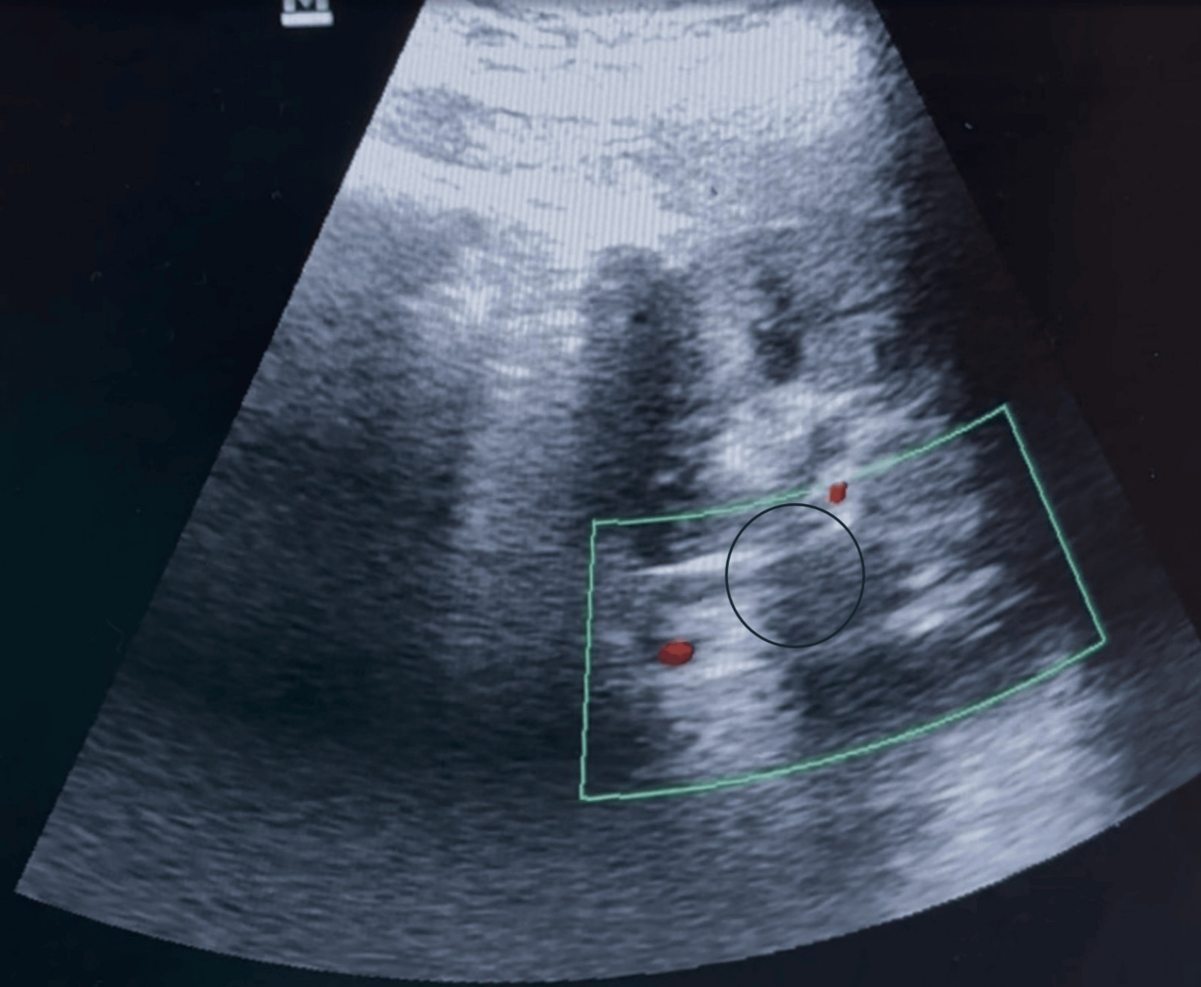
Renal Doppler image showing lumen occluding thrombus in the proximal left renal vein
